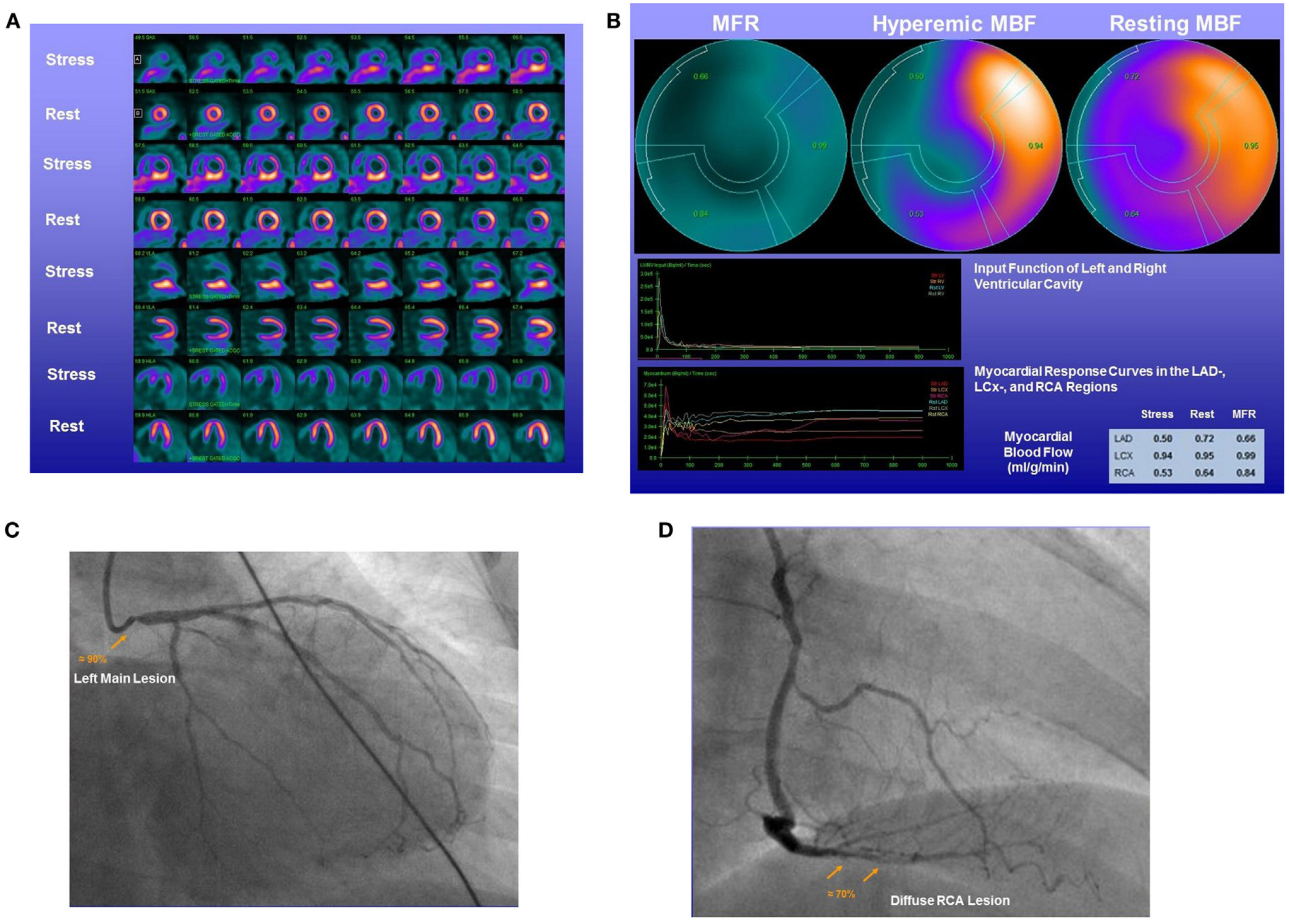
^13^N-ammonia positron emission tomography/computed tomography in the assessment of complex CAD. A 58-year-old women with type 2 diabetes mellitus and dyslipidemia presented with effort-induced angina symptoms. (A) On stress ^13^N-ammonia perfusion images, there is a severe decrease in radiotracer uptake of the anterior, anteroseptal, apical, and less severe in the anterolateral regions of the left ventricle, which becomes reversible on the rest images to signify large-sized, severe ischemia. On stress images, the inferior wall demonstrates pronounced radiotracer uptake likely due to overlapping high liver uptake that hampers an accurate evaluation. (B) Quantification of myocardial blood flows (MBFs) demonstrates a decrease of hyperemic MBFs from rest in all three major coronary artery territories of the left anterior descending artery (LAD)-, left circumflex artery (LCx)-, and right coronary artery (RCA) distribution. This resulted in regionally reduced myocardial flow reserves (MFRs) of 0.66 in the LAD-, 0.99 in the LCx-, and 0.84 in the RCA distribution, respectively. (C) Invasive coronary angiography signifies a high-grade lesion of the left main stem. (D) In addition, a diffuse ≈70% lesions in the distal RCA are noted. Consequently, the distinct reduction in MFR in the RCA distribution also unmasks the hemodynamic significance of the mid RCA lesions, apart from the left main, and the patient was referred for surgical revascularization of both arteries.
